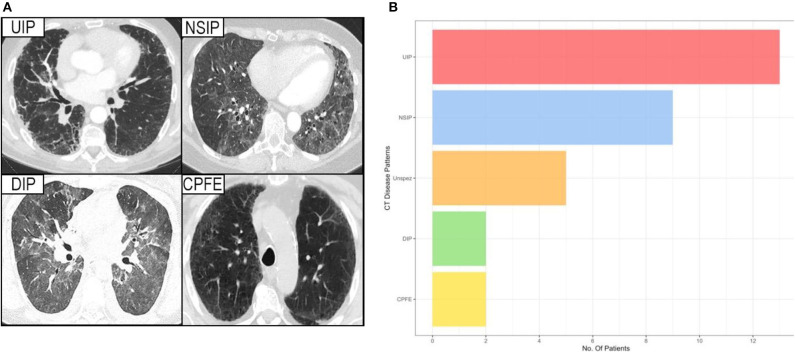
(A) Showing the HRCT pattern of the various interstitial lung diseases. (B) Showing the prevalence of different forms of interstitial lung disease among patients with a proven Sjögren's syndrome, considered contributory to their lung disease. UIP, usual interstitial pneumonia; NSIP, non-specific interstitial pneumonia; DIP, desquamative interstitial pneumonia; CPFE, combined pulmonary fibrosis and emphysema; Unspez, unspecific interstitial changes.
